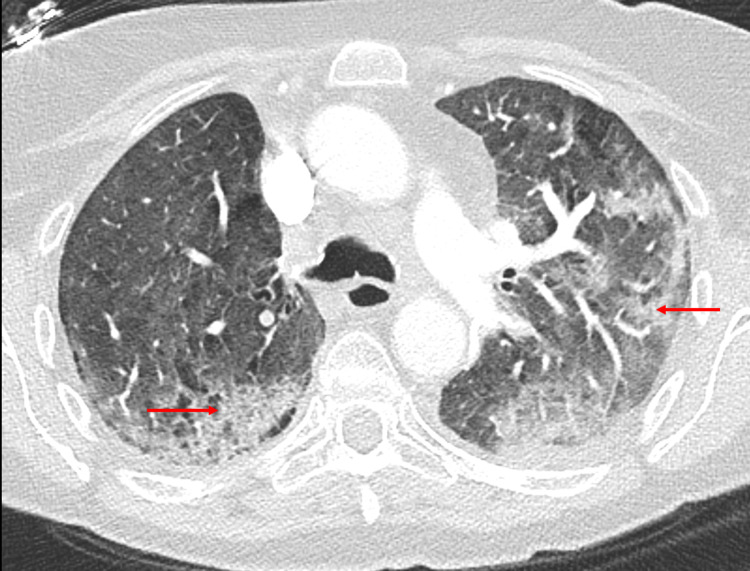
Chest CT pulmonary embolism protocol revealed new multifocal ground-glass interval consolidative opacities (red arrows), interlobular septal thickening, bilateral pleural effusions, and no pulmonary embolism.
